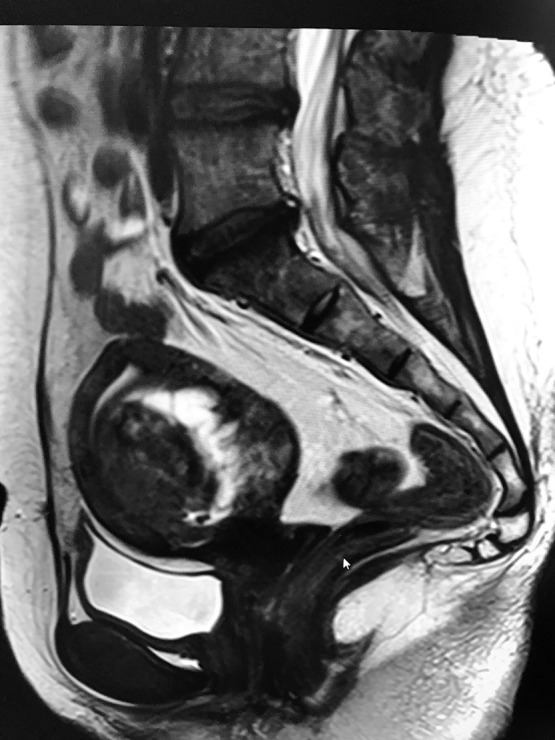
Magnetic resonance imaging (MRI) showing a solid, 60-×50-mm mass with a cystic component within the uterine cavity.
